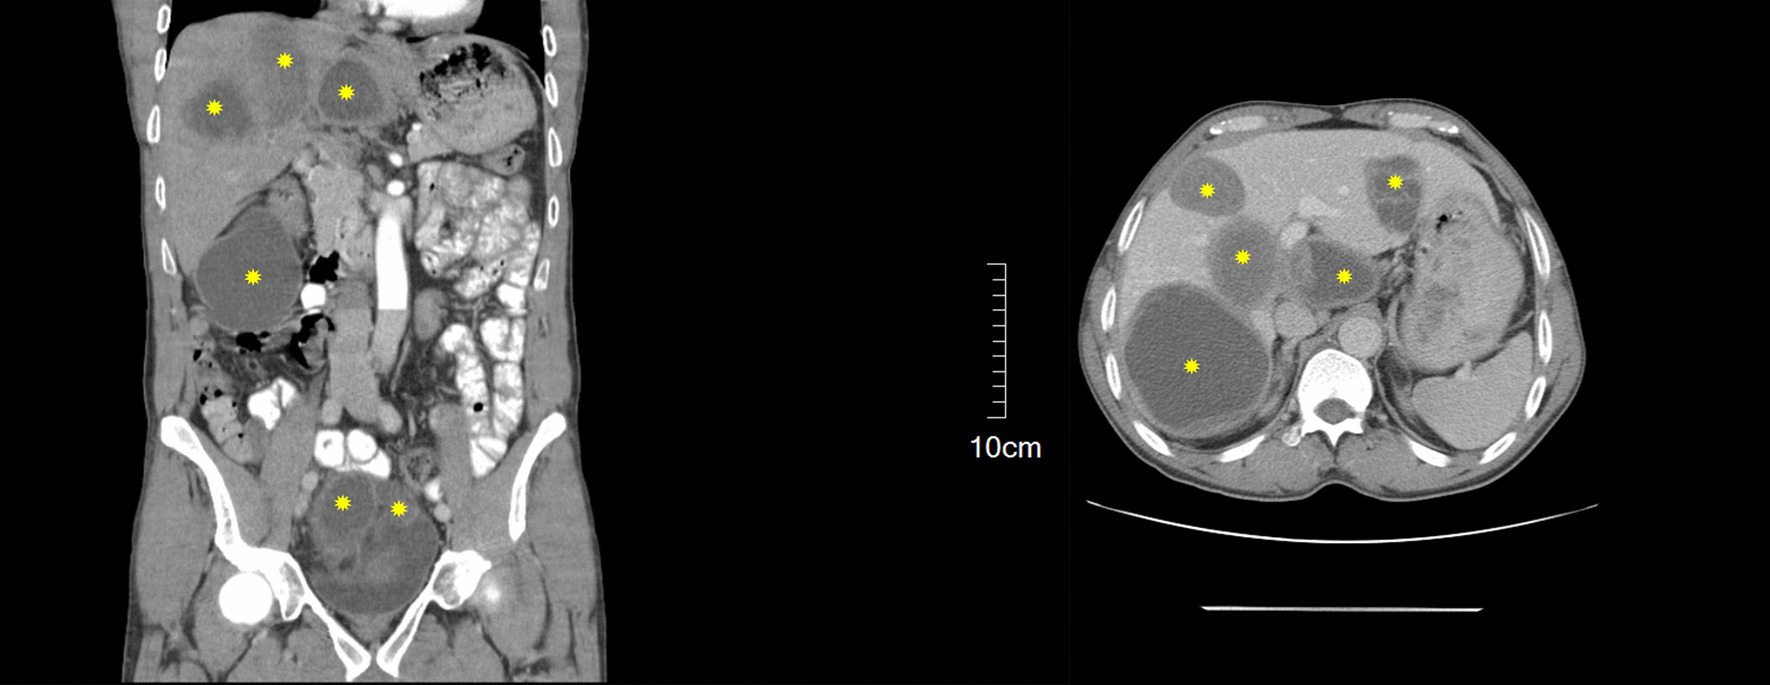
Abdominal CT scan showing multiple cystic lesions in the liver, subhepatic region, omentum, and pelvis (yellow stars)
